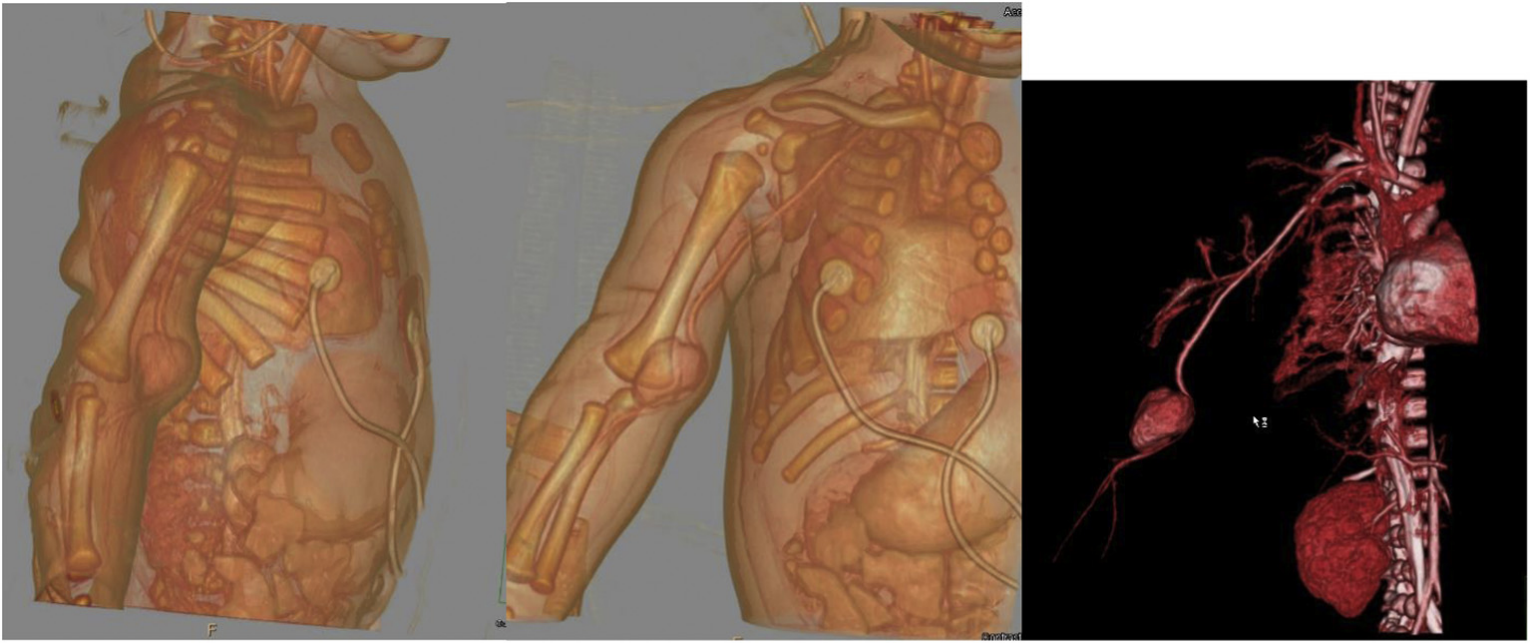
Three-dimensional computed tomography reconstruction of the right distal brachial artery pseudoaneurysm measuring 1.8 × 1.6 × 2.1 cm. The pseudoaneurysm communicated with the brachial artery and had contrast opacification of its lumen. No filling defect was seen within the pseudoaneurysm to suggest the presence of thrombus.
